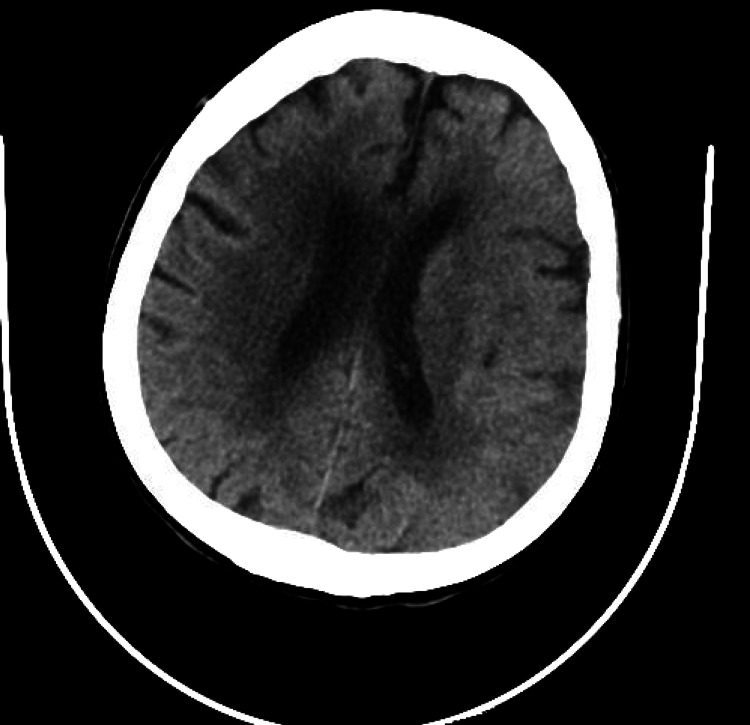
CT scan of the head negative for intracranial bleed or acute infarct.
